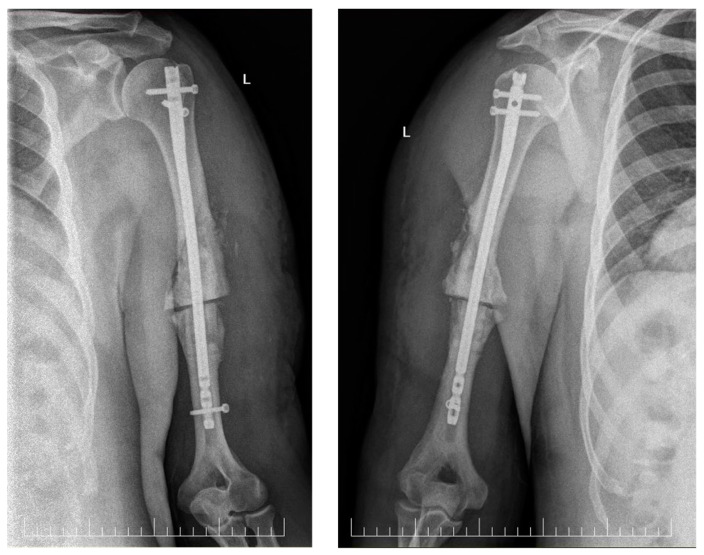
Postoperative X-ray of the left humerus. Left: antero-posterior view, and right: latero-lateral view, showing the IMN fixation.
